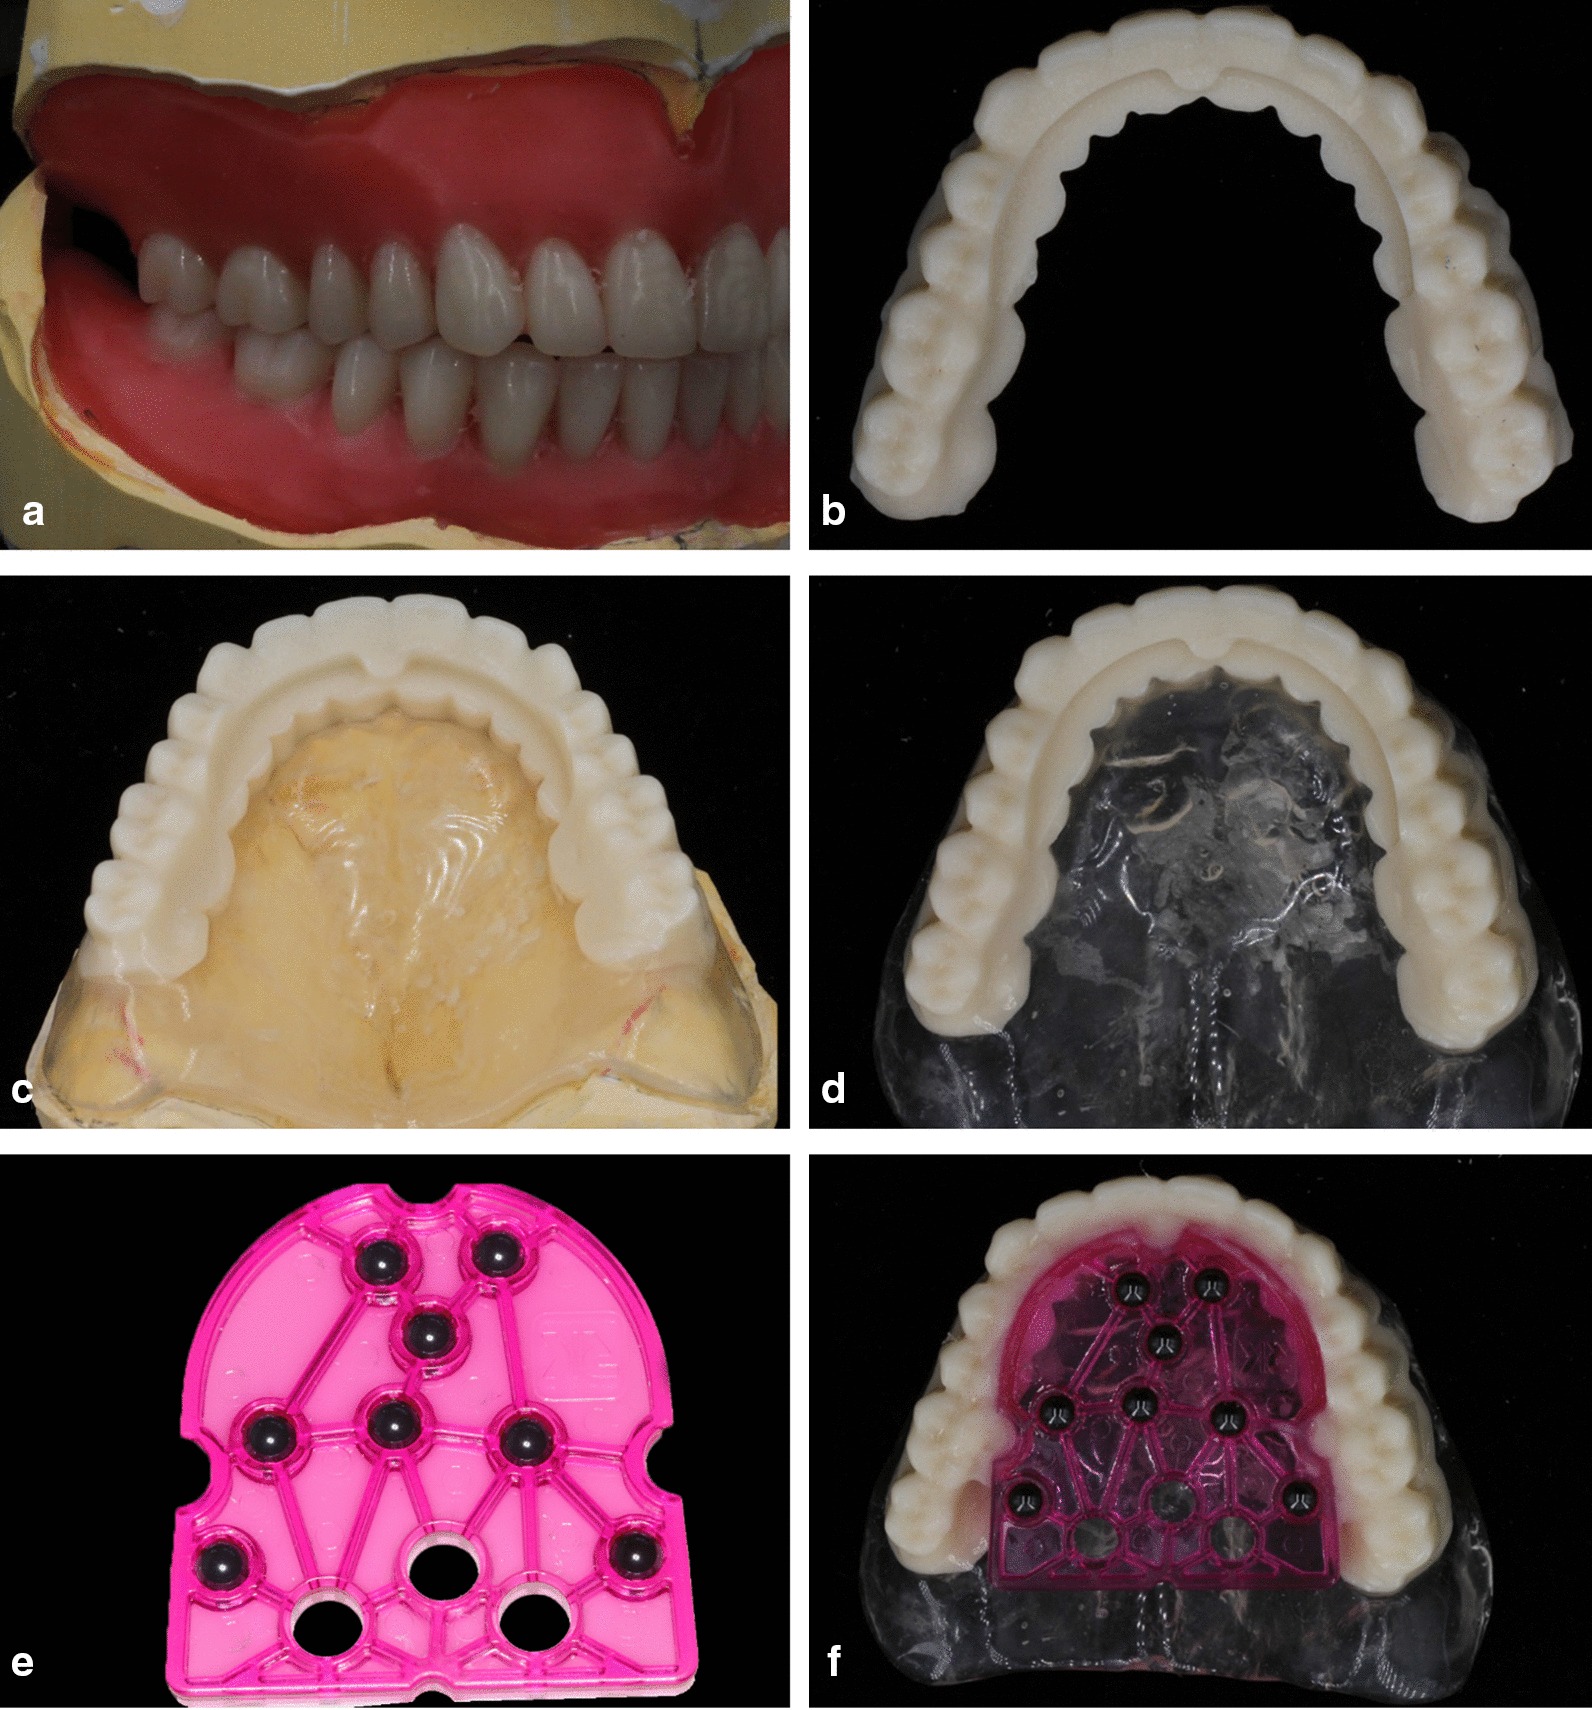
The radiographic template. a The wax-up on the maxillary and mandibular edentulous models. b Radiopaque PMMA dentition duplicated from the digitized wax-up. c Translucent resin base for radiopaque dentition built on the stone model. d Radiopaque dentition with the transparent resin base removed from the stone model. e Diagnostic template with eight zirconia beads. f The finished radiographic template
